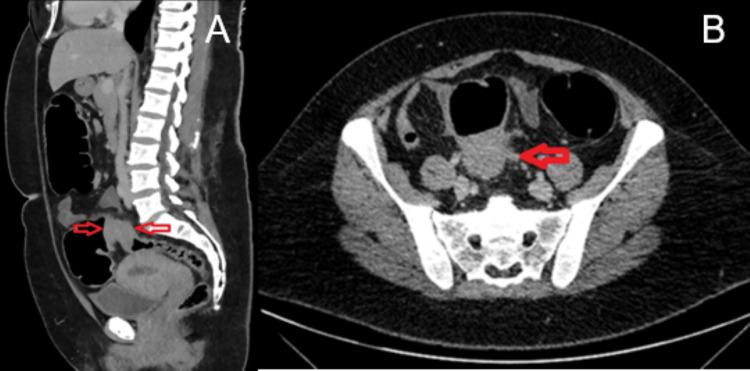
Sagittal CT scan of the abdomen and pelvis (A) demonstrating an obstructing mass at the sigmoid colon with thickening of the bowel wall and irregularity of the serosal surface (red arrows). Axial CT scan of the abdomen and pelvis (B) demonstrating small nodules within the adjacent mesentery of the sigmoid colon (red arrow).
